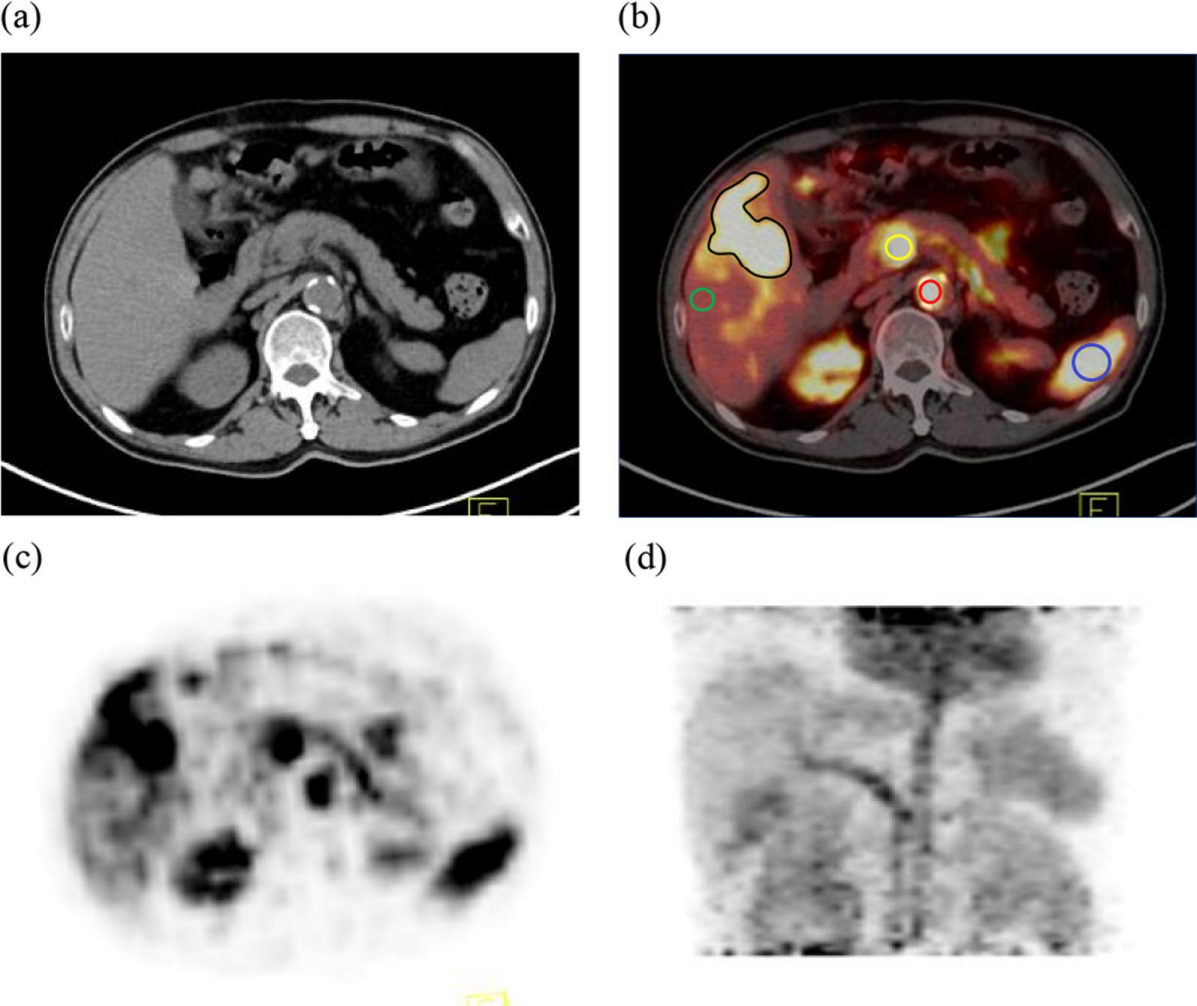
Delineating regions of interest (ROIs) in dynamic PET/CT. a CT image, (b) PET/CT image, (c) PET image, and (d) maximum density projection image. ROIs are manually drawn, and HCC is shown in black, background liver tissues in green, aorta in red, portal vein in yellow and spleen in blue
